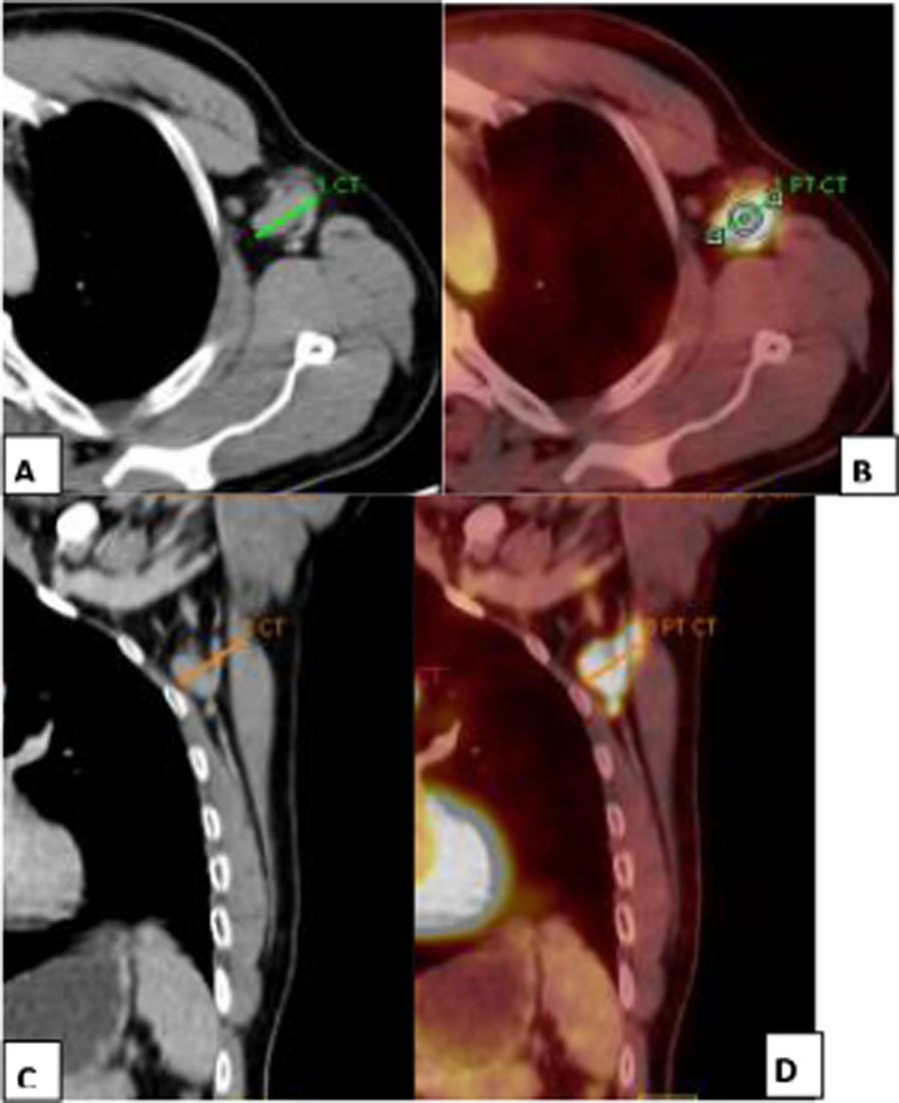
A 39-year-old male patient with NHL, treated with anti-cd20 antibody rituximab 3 months prior to vaccination. FDG PET/CT performed 7 days after the 2nd dose of Pfizer-BioNTech COVID-19 vaccine. A Axial and C coronal CECT images at the axillary level revealed enlarged left axillary LNs about 3.5 cm B Axial and D coronal PET/CT images revealed FDG-avid left axillary LNs with 3.7 SUVmax uptake
